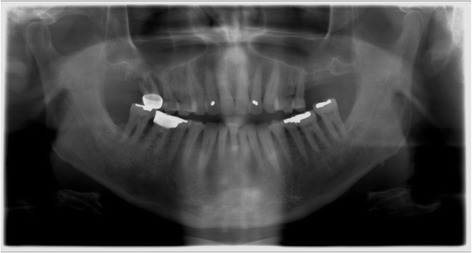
Initial presentation. Panoramic radiograph taken at initial visit shows severe bone loss, supraerupted molars and furcation involvement
